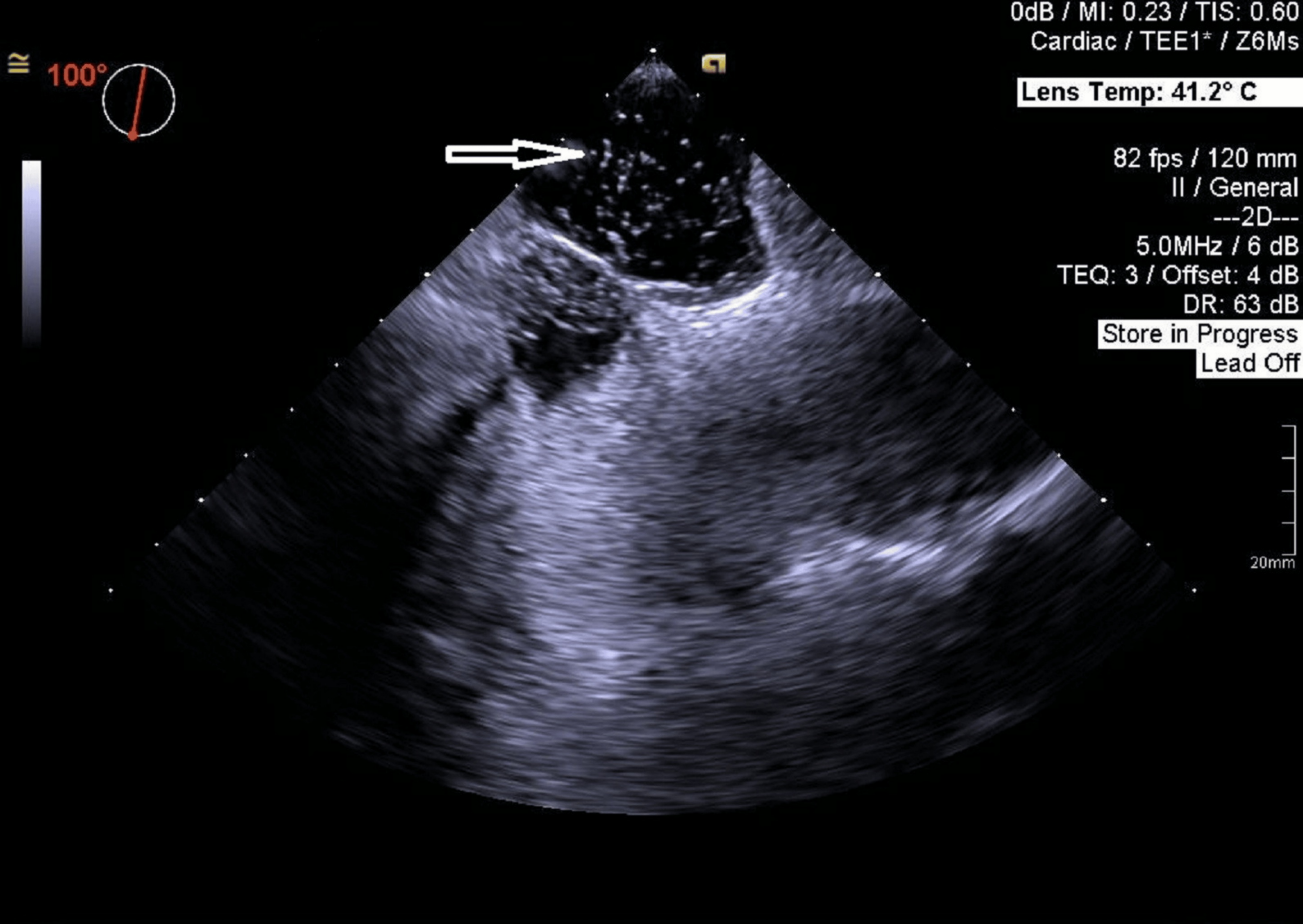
TEE showing bubbles crossing the PFO after two cardiac cycles. TEE: transesophageal echocardiography; PFO: patent foramen ovale.
